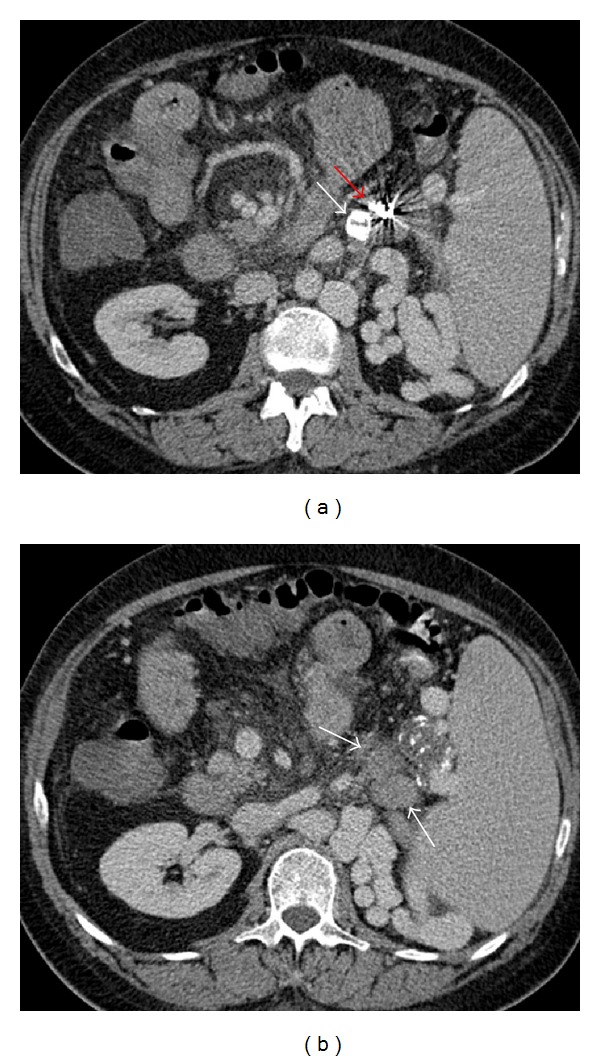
(a) Contrast-enhanced CT on postprocedure day 2 demonstrates Amplatzer vascular plug (white arrow) and coils (red arrow) within a splenorenal shunt. (b) White arrows demonstrate absence of flow distally in embolized splenorenal shunt.
